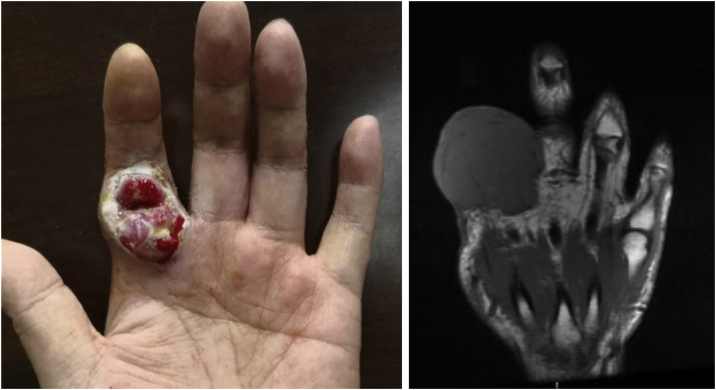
Clinical images of skin lesion, July 2020. (A) Red-purple painless nodule in the left index finger. (B) A representative MRI image
